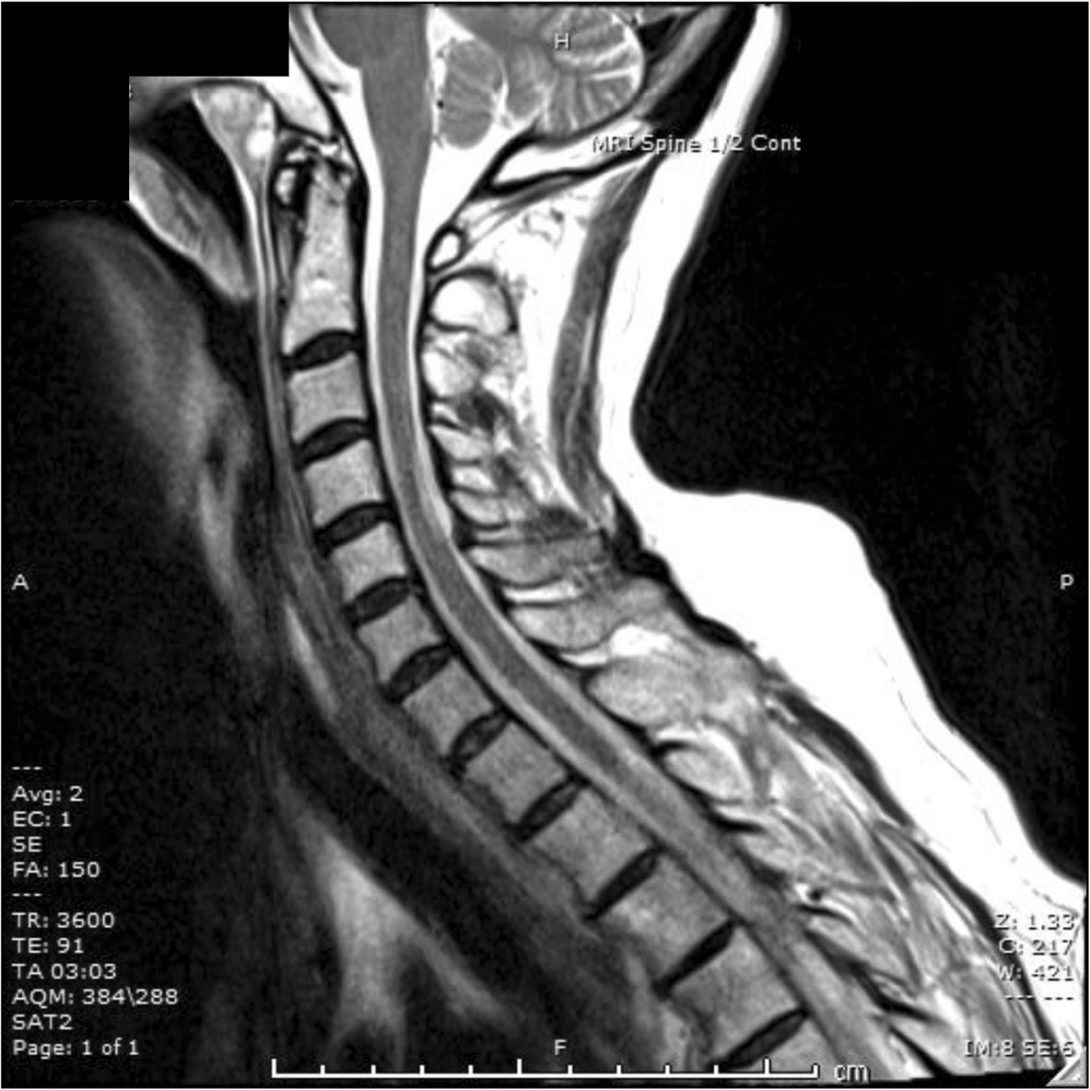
T2-weighted magnetic resonance imaging (MRI) of the spine demonstrating abnormal high T2 signal involving the dorsal column of the cervical cord
